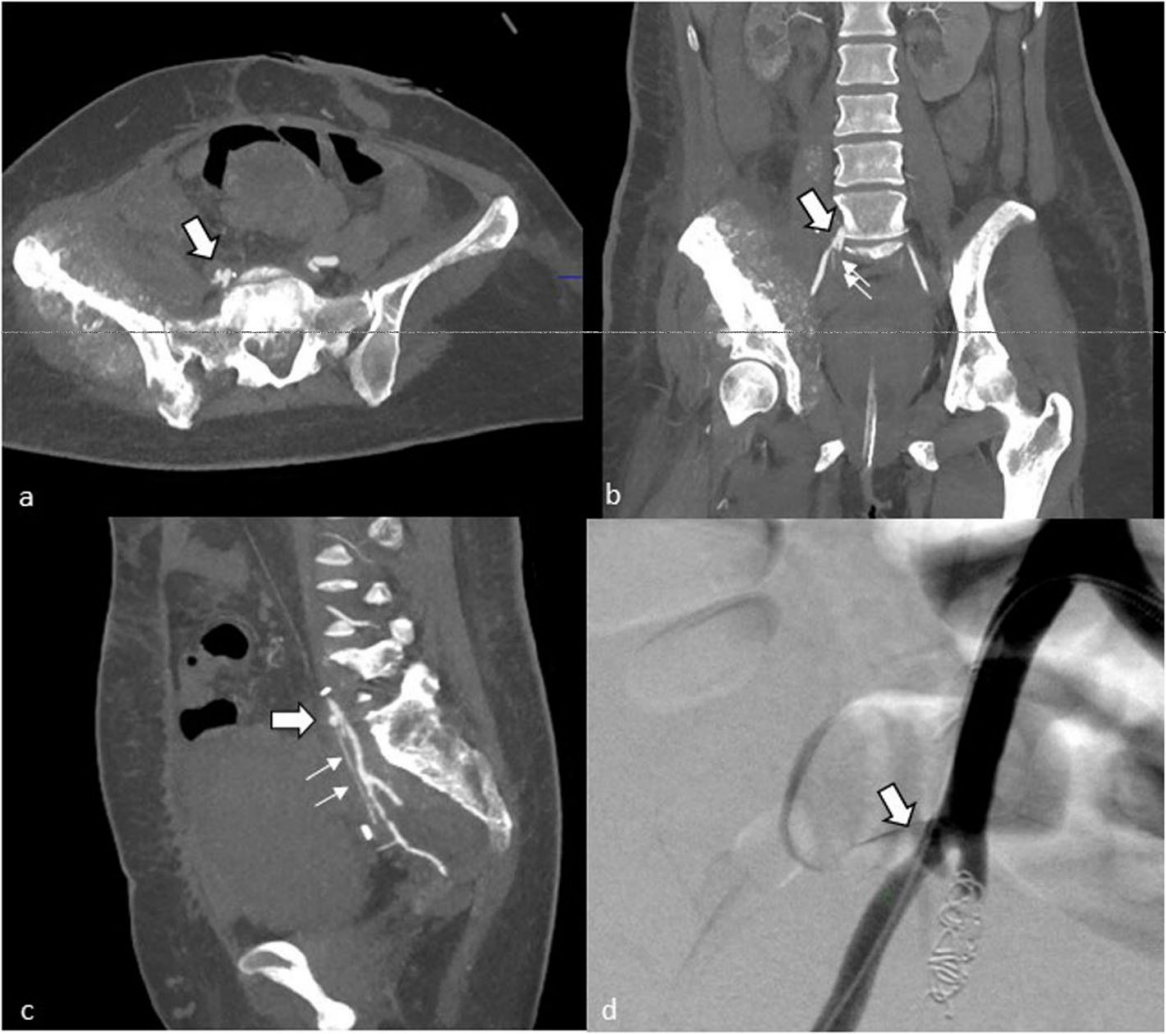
Case #1: Contrast enhanced computed tomography confirmed the ureteroarterial fistula (large arrows) and demonstrated even extravasation into the right ureter (small arrows) (a, b, c). Angiography shows a defect at the iliac junction (large arrow). Coil embolization of the internal iliac artery has already been performed (d)
